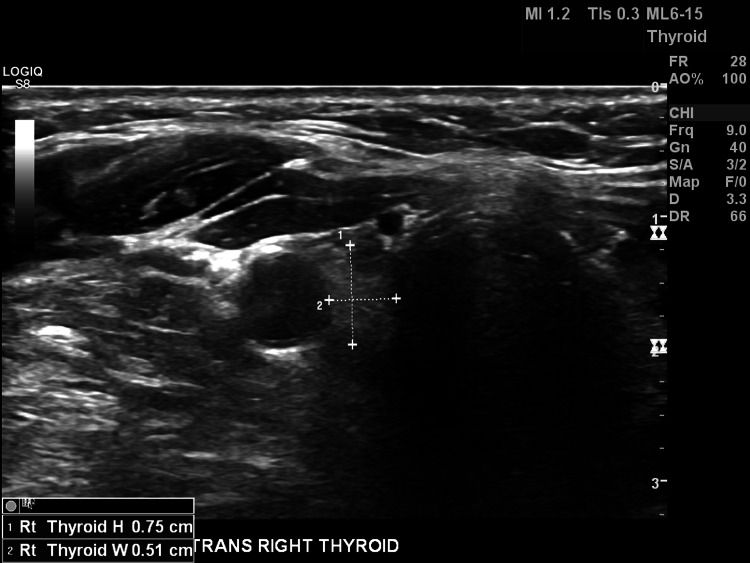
The ultrasound shows only the right lobe of the thyroid is present.
